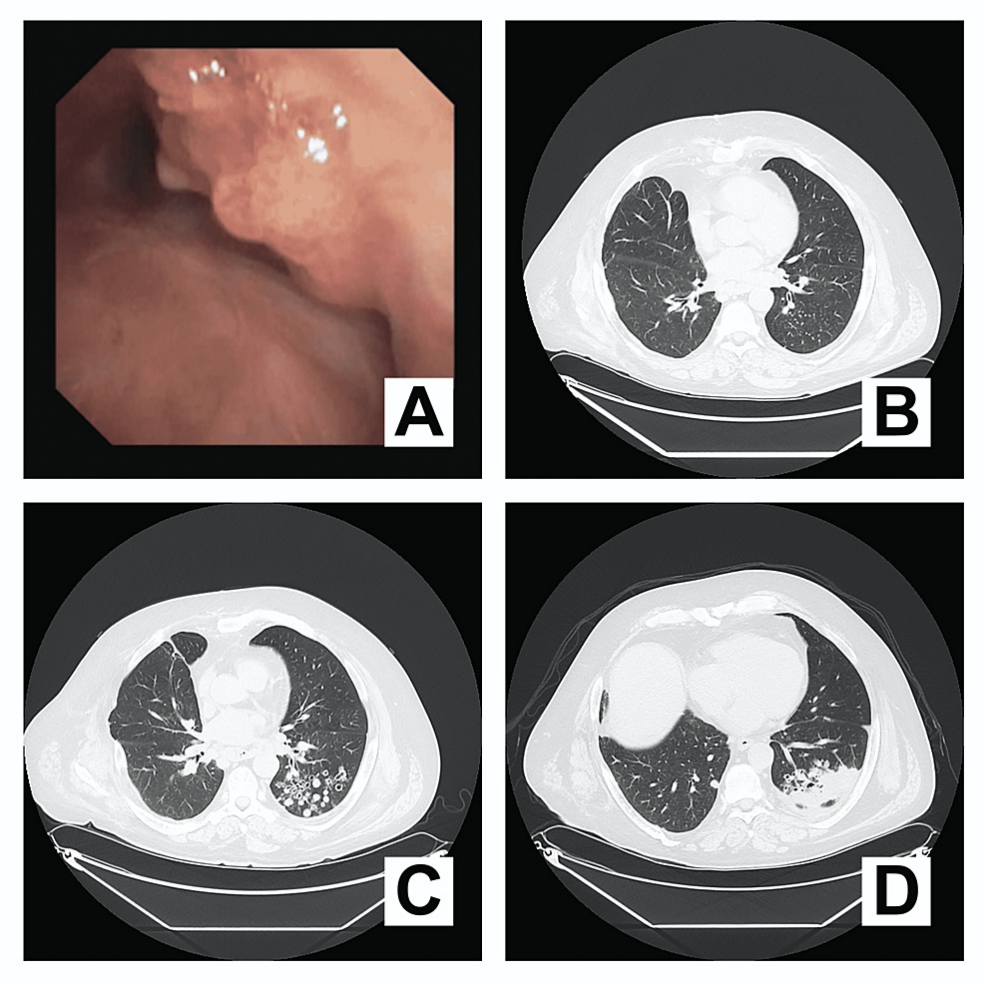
Papillomas in the trachea (A). CT scans showing bilateral small lung nodules (B), progression to cavitary nodules (C), and progression to squamous cell carcinoma (confirmed by biopsy) (D).
